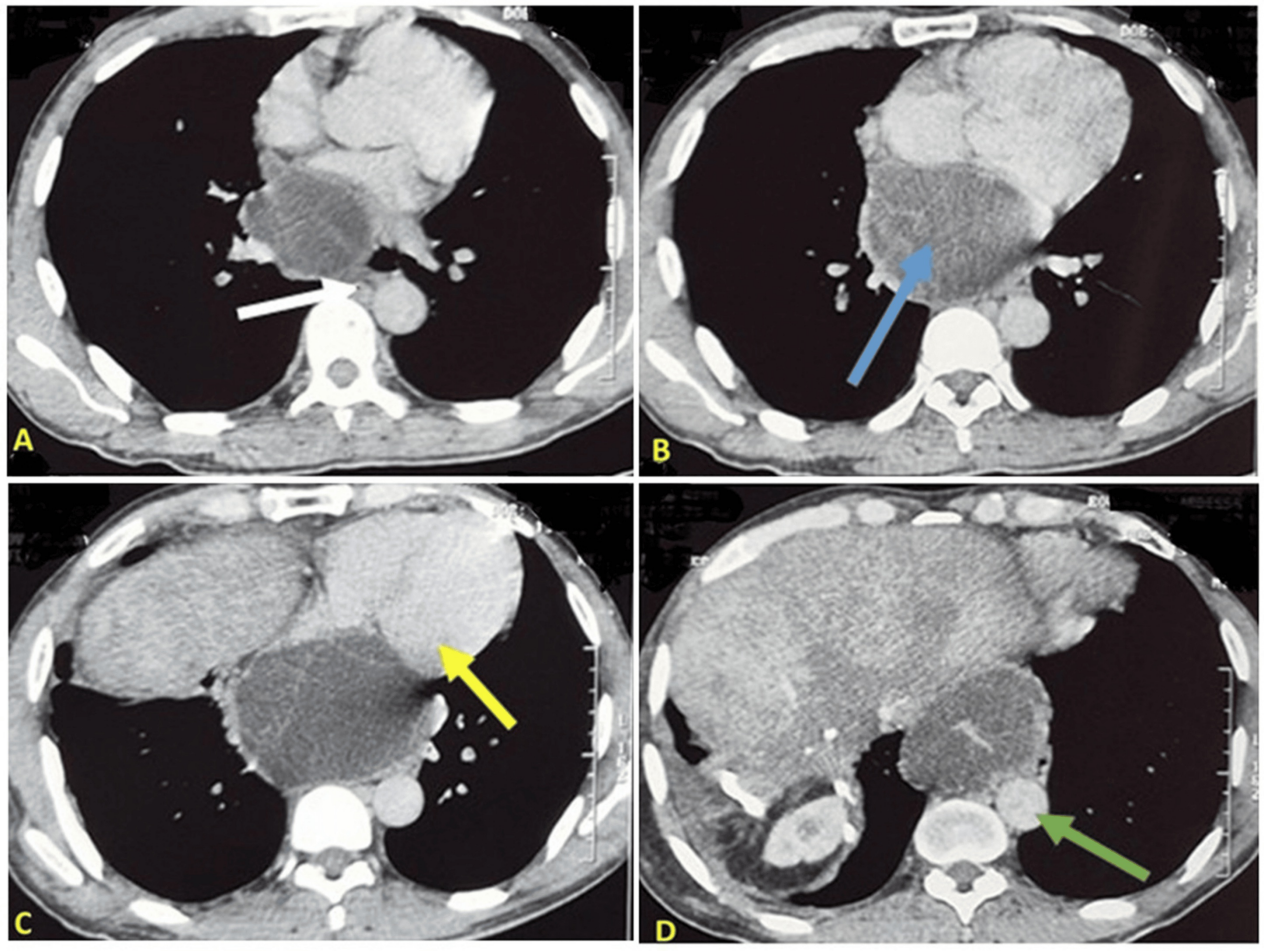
Thoracic CT scan: Image B shows a well-defined hydatid cyst located in the inferior retrocardiac mediastinum (blue arrow), measuring 9.3 × 7.0 cm. The lesion displaces and compresses adjacent mediastinal structures, including the atria (Image C, yellow arrow), thoracic aorta (Image D, green arrow), and esophagus (Image A, white arrow).
